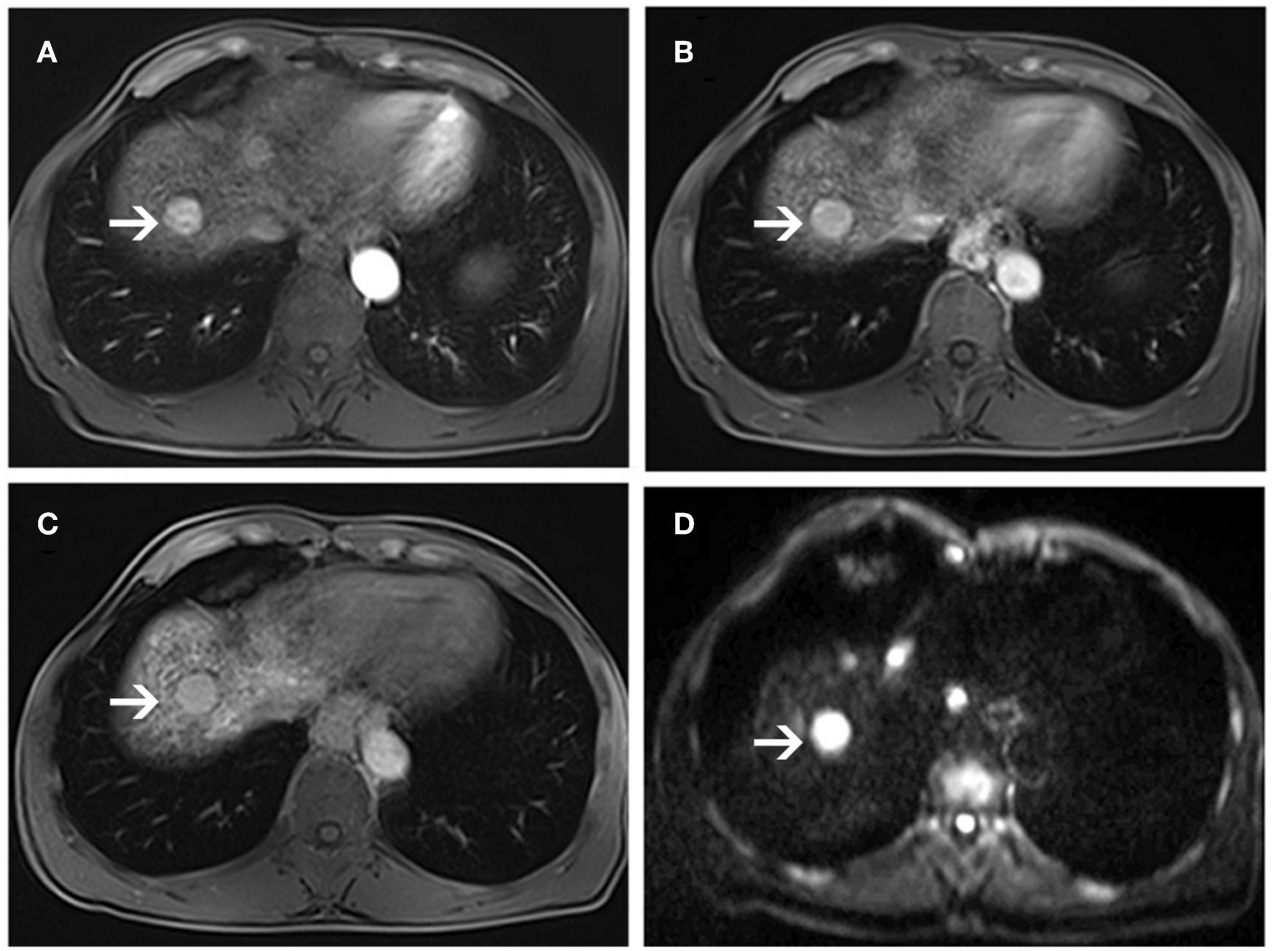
MR images of a 56-year-old man with a pathologically proven high-grade dysplastic nodule (white arrows) and a history of hepatitis B virus infection. Arterial-phase image (A) shows an enhancing nodule in segment VIII of the liver. Equilibrium-phase MR image (B) shows a nodule not demonstrating washout of the contrast material. A hepatobiliary phase image (C) shows nearly isointensity compared to the surrounding liver parenchyma. On diffusion-weighted image (D), the lesion shows hyperintensity compared to the surrounding liver parenchyma.
